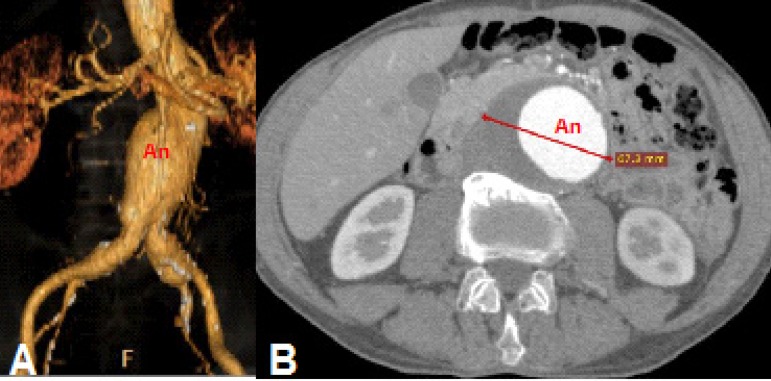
Case 4: computed tomography (CT) angiography of abdominal aortic
